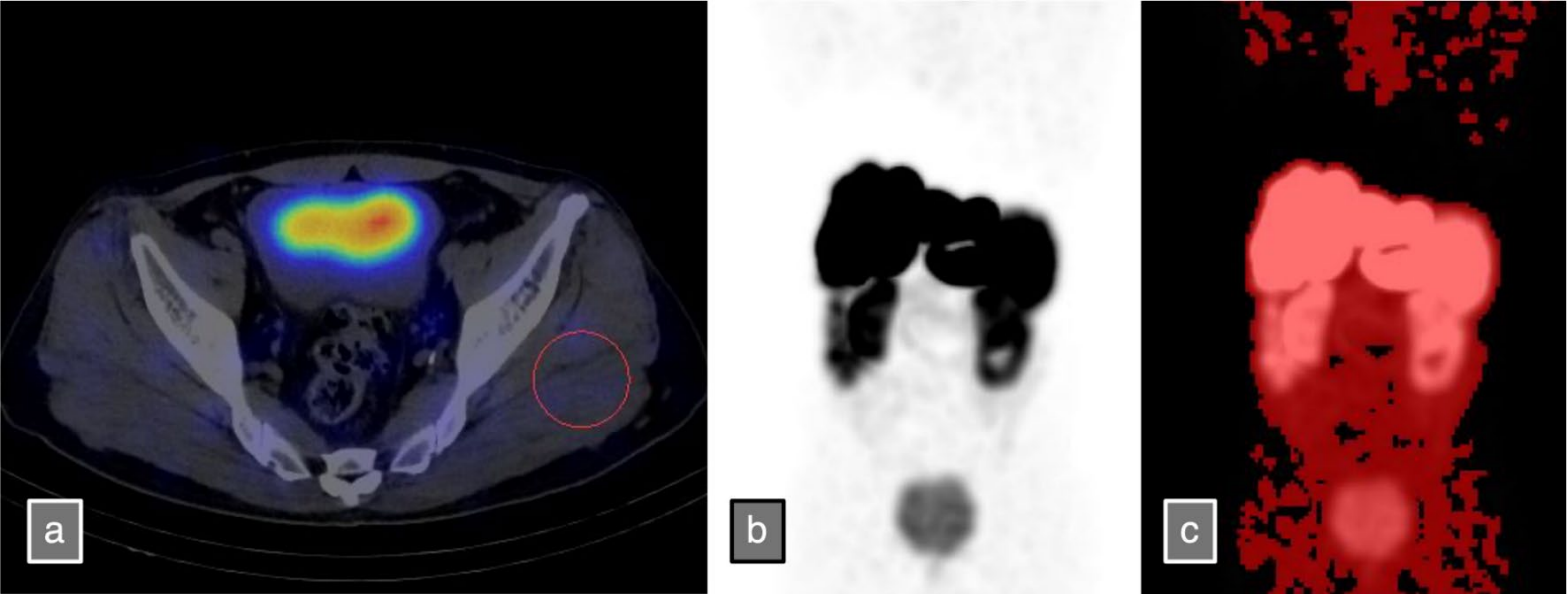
a. A case of a male in his 50 s with a pancreatic primary neuroendocrine tumor and multiple liver metastases. A [^177^Lu] DOTATATE SPECT/CT fusion image is presented. Spherical VOIs with a diameter of up to 5 cm were placed on the skeletal muscles of the gluteal region (gluteus maximus and gluteus medius), and the SUVmean and standard deviation were measured. b. [^177^Lu] DOTATATE SPECT MIP image. c. Using the open-source software Metavol, all regions with SUVmean + 2SD or higher of the skeletal muscles were set as VOIs, and LUTtotal was measured. The measured SUVmean was 0.088 with an SD of 0.059, resulting in a threshold of SUVmean + 2SD at 0.206. The SUVmean of the entire region exceeding this threshold was 3.60, and the LUTtotal was calculated as 31,062 by multiplying this value by the VOI volume. Since the total amount of RI in the body is considered to influence the external dose rate, the VOI includes not only tumor uptake but also physiological uptake in the kidneys and spleen, as well as urinary accumulation in the urinary tract. SPECT/CT, single-photon emission computed tomography; VOI, volume of interest; SUV, standardized uptake value; MIP, maximum intensity projection; LUTtotal, defined as the product of the VOI volume and its SUVmean
